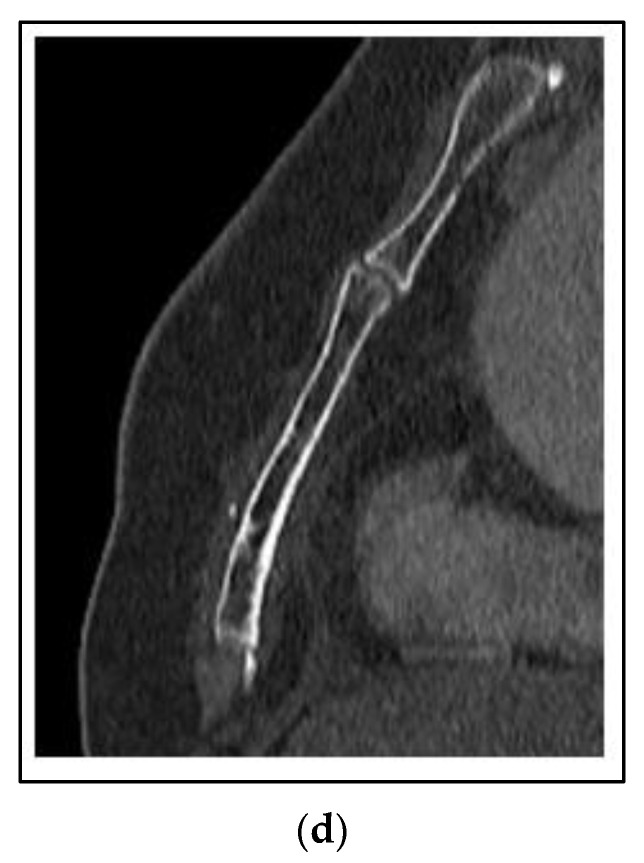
Case 1: Simultaneous oligometastases. A 60-year-old woman presents with a locally advanced cancer of the left breast cT1N3M1 with an oligometastasis of the sternum. The sternal oligometastasis is shown on representative images from CT (a) and bone scan (b) imaging. (c) Radiation therapy to the left breast and sternum. The left image shows a colorwash image superimposed on a radiation planning CT of the dose from the sternum-directed SBRT given 40 Gy in 5 fractions, and the right image shows the colorwash of the dose of 50 Gy given to the breast and regional nodes combined with the sternal treatment. The yellow color indicates the prescribed dose of radiation, and the green and blue colors around the edges indicate the medium and lower dose fall-off of the radiation dose plan (d) The appearance on a CT scan image of the sternum 3 years after treatment. There is minimal residual sclerosis of the sternum with no evidence of progression.
